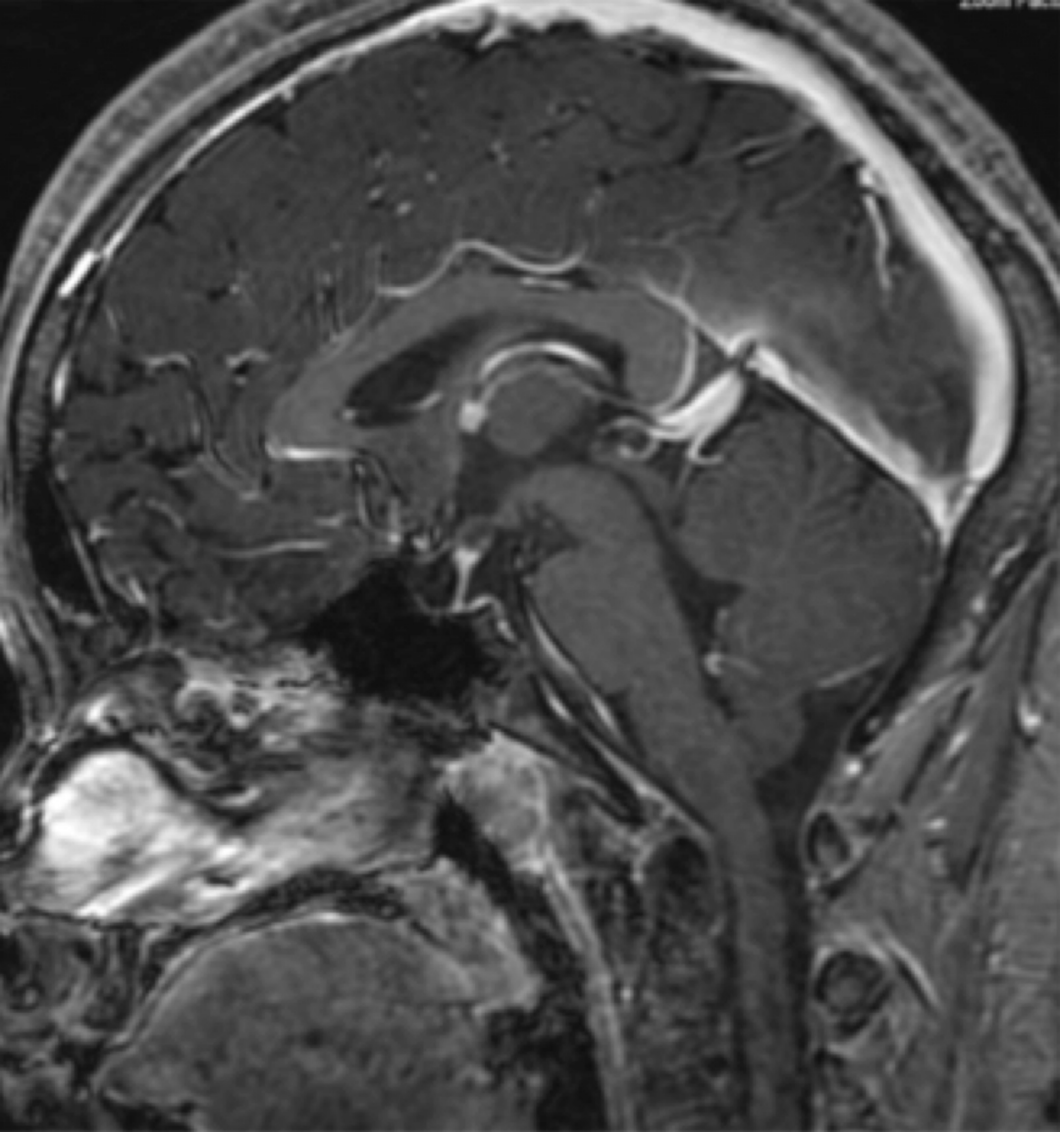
Post-treatment MRI Scan Sagittal T1 post-contrast MRI 32 months after diagnosis indicates no abnormalities.
